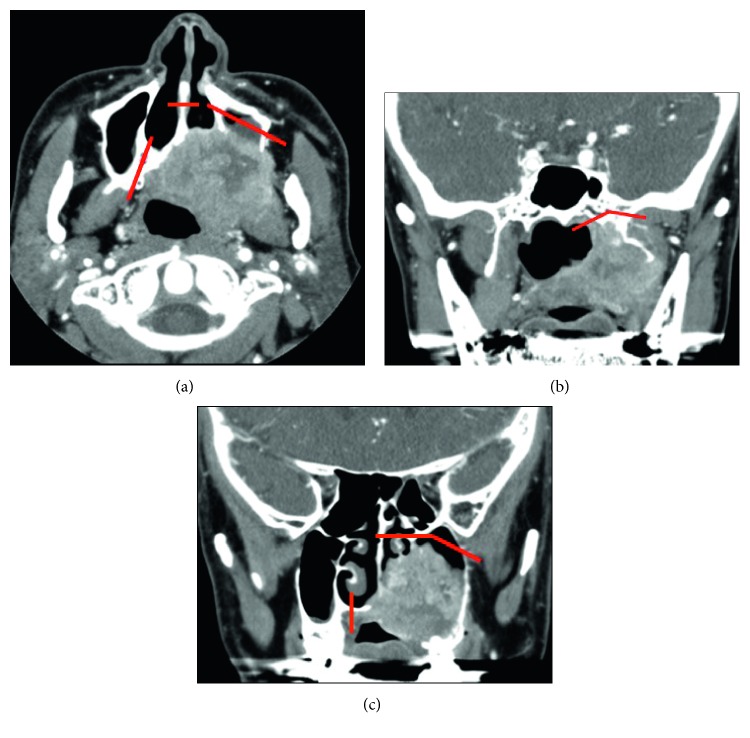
CT views. (a) Axial view: tumor invading the left posterior maxillary sinus but not the anterior area. (b) Coronal view: tumor invading the base of the pterygoid process. (c) Tumor not invading the orbital fossa or cranial bone. Red lines are dissection lines.
